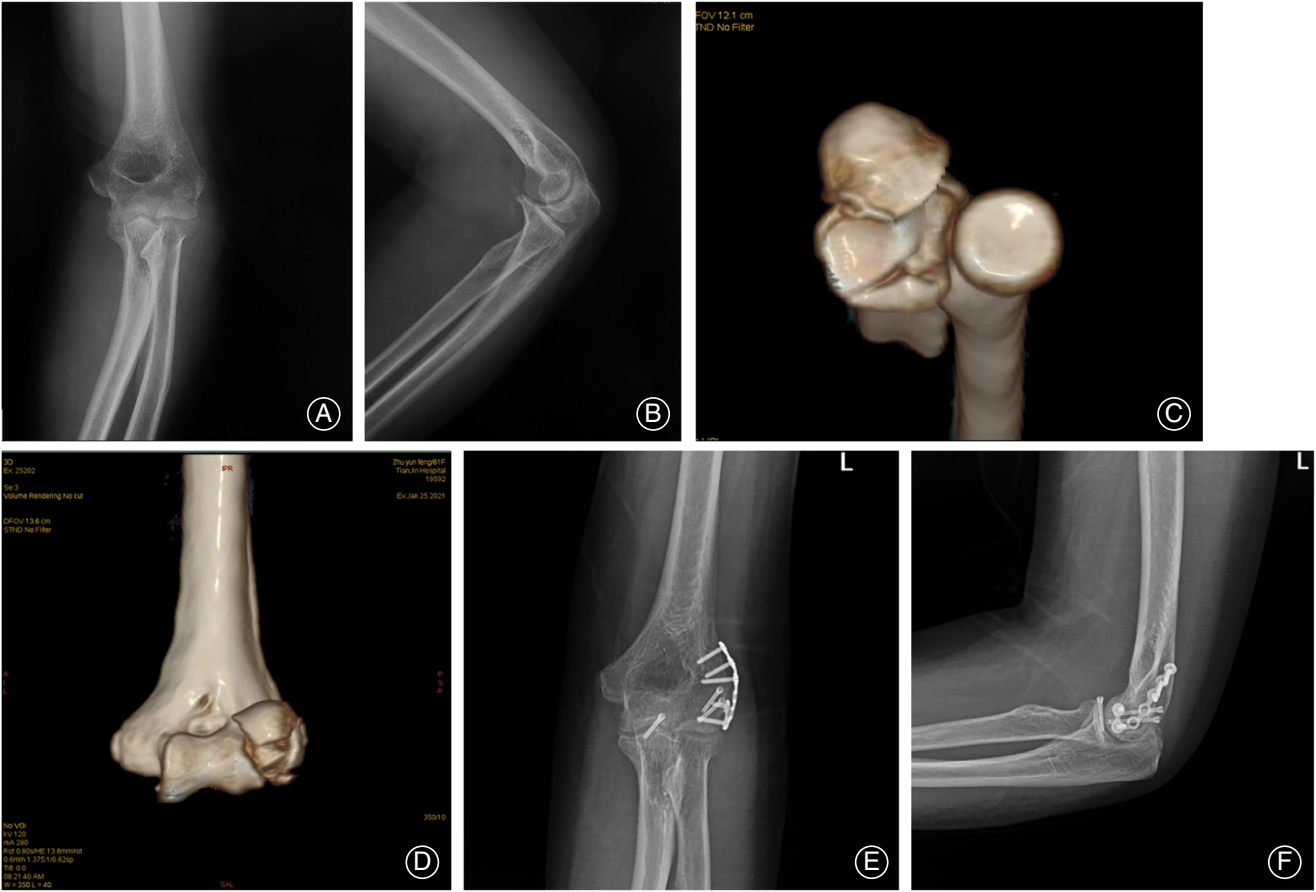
Patient No. 16, the only case combined with capitellum fracture in this study. A 61‐year‐old woman fell from the standing height and injured her left non‐dominant elbow. (A, B) Initial anteroposterior and the lateral radiograph showed a suspected fracture of the coronoid process of the ulna. (C, D) A computed tomography image showed an Adams Type IV(AL)a fracture of the coronoid process and the capitellum fracture. (E, F) The anteroposterior and lateral radiographs that were obtained following the operative treatment.
